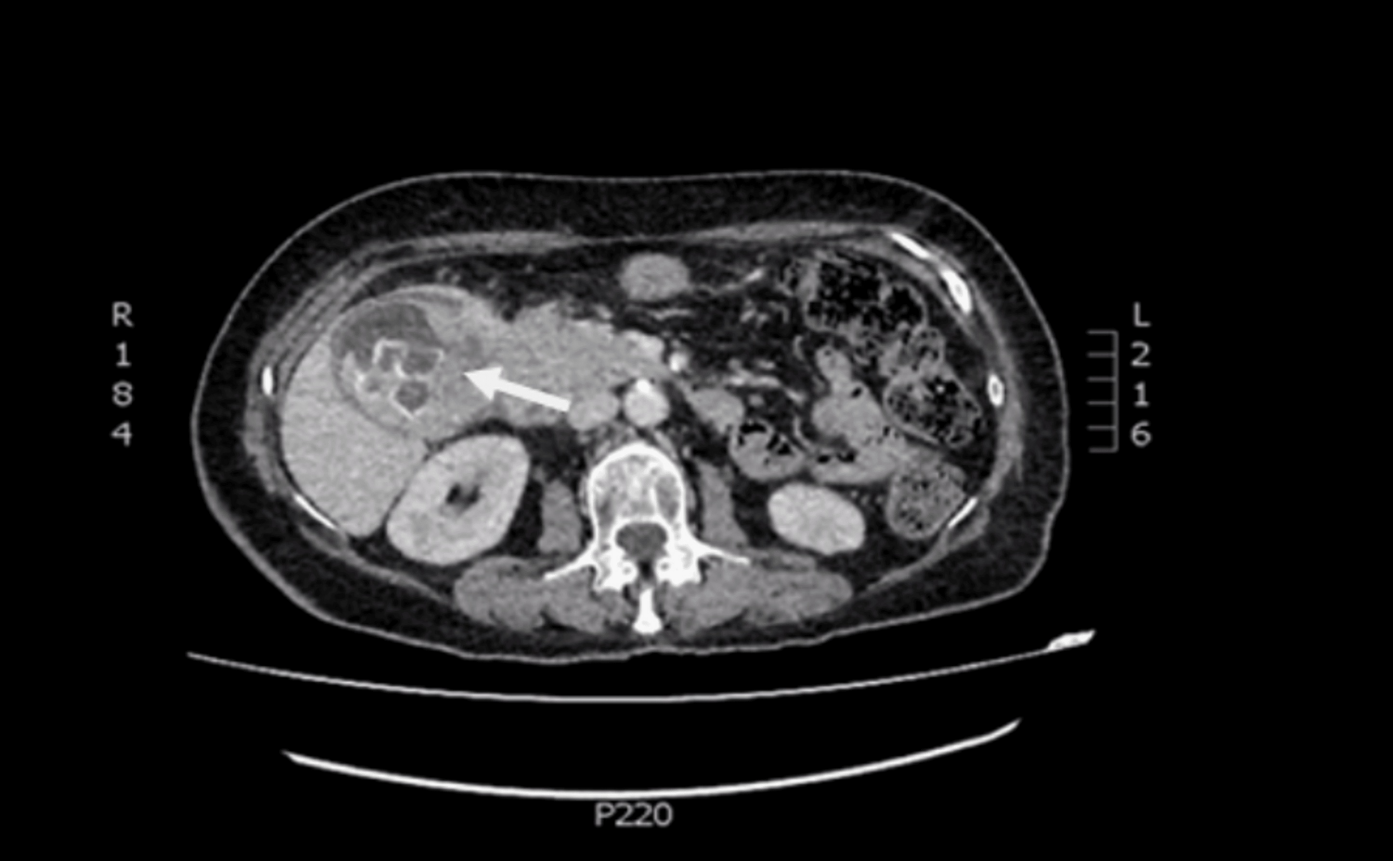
Coronal contrast-enhanced CT image of the abdomen demonstrates gallbladder mass lesion and gallbladder stones CT: computed tomography
